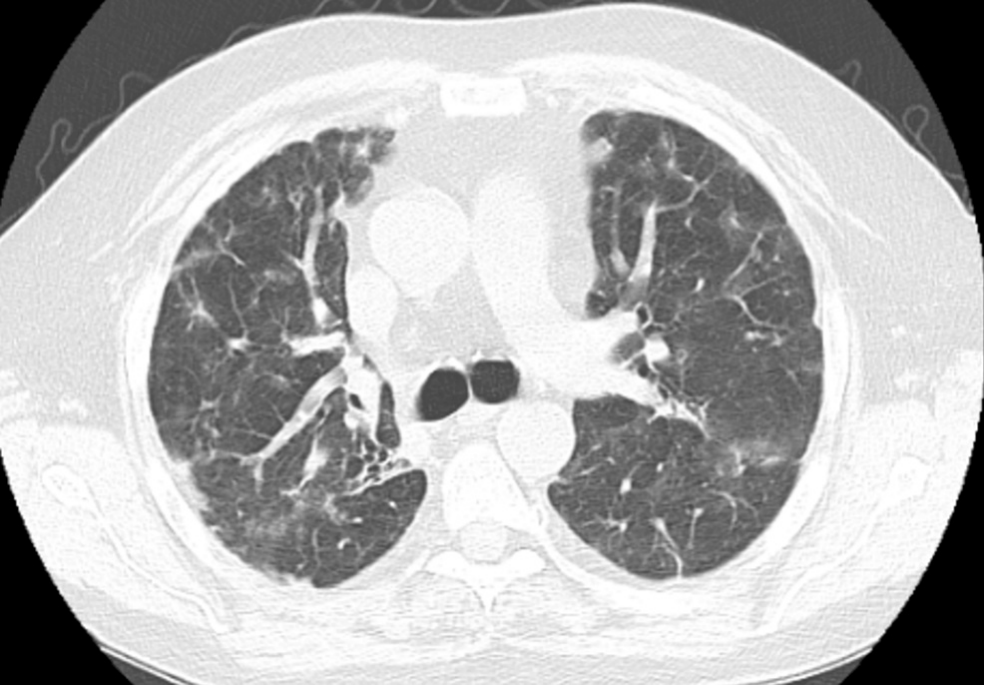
Follow-up CT chest with contrast two months after initiation of steroid therapy demonstrated marked improvement of the previously noted confluent and nodular opacities.
